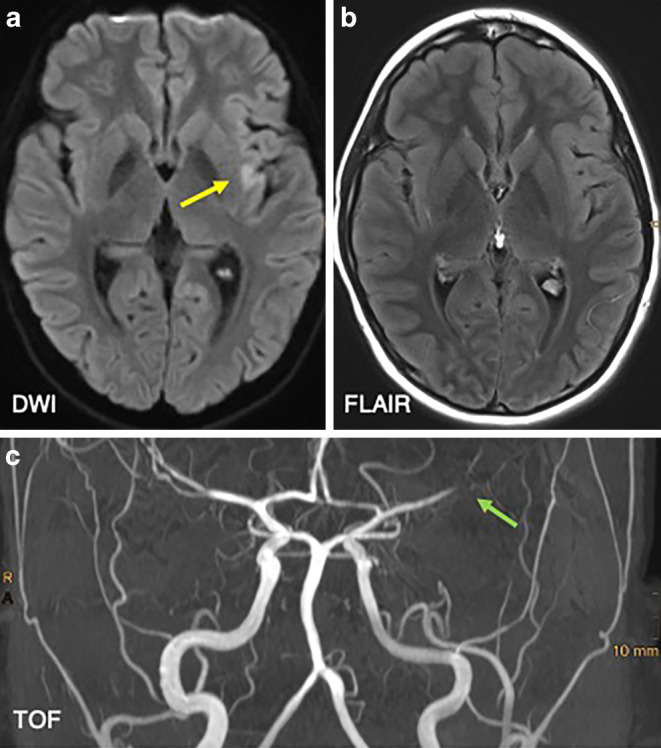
MRI scan of a 13-year-old boy presenting with headache, right arm paresis, and facial palsy. MRI with diffusion-weighted imaging (DWI) (b = 1000 s/mm2, axial view; (a) and FLAIR (axial view; b) demonstrated a lesion with restricted diffusion of the left insular cortex (yellow arrow) without correlate on FLAIR images. Maximum intensity projection (MIP) images from TOF angiography (c) showed a left distal M1 stenosis (green arrow) with flow restriction
